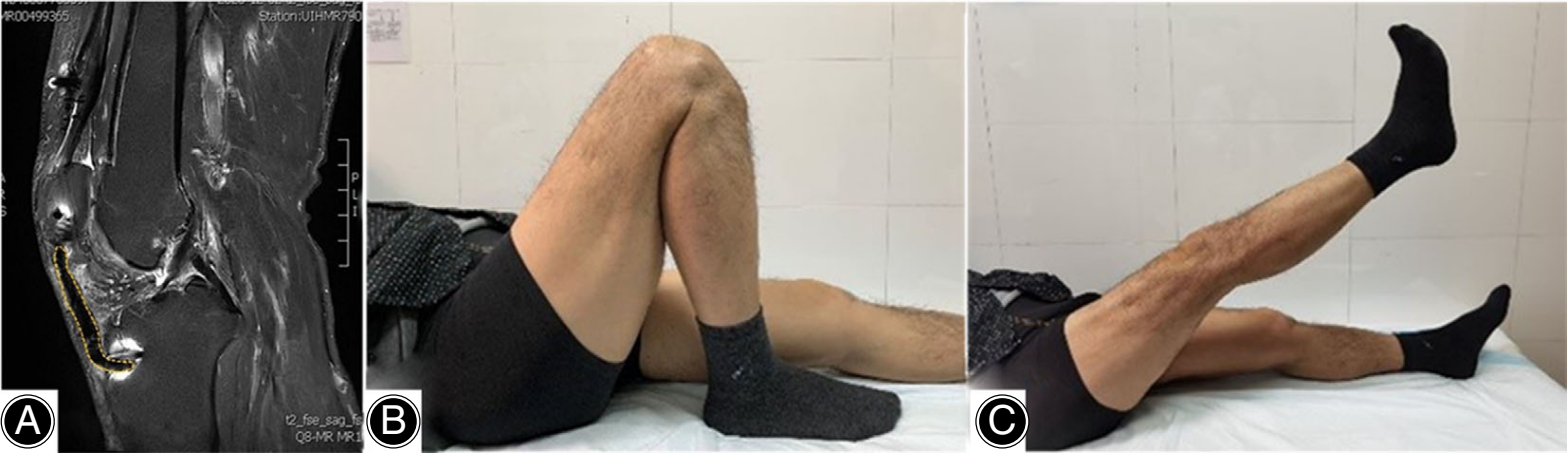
At 12 months follow‐up: (A) MRI of the right knee showing patellar tendon‐like structure on the sagittal plane (yellow dashed line); Postoperative range of motion of knee joint: knee flexion (B) and knee extension (C).
